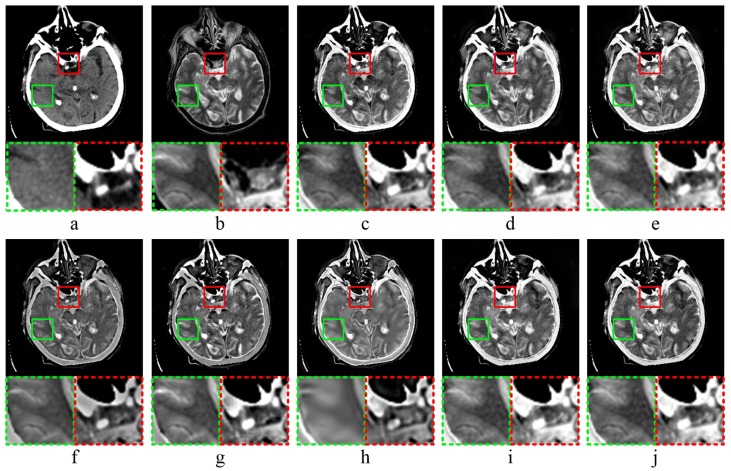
MR-CT image fusion experiments: (a,b) source images; and (c–j) the fused image obtained by MST-SR, NSCT-PC, NSST-PCNN, ASR, CT, KIM, CNN-LIU, and the proposed method, respectively. Two partially enlarged images marked in green and red dashed frames correspond to the regions surrounded by green and red frames in the fused image.
